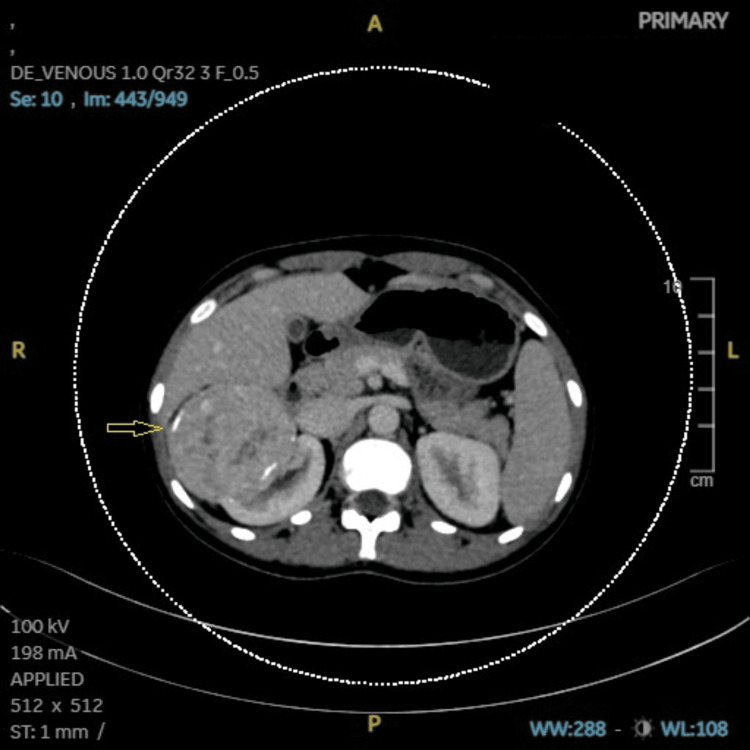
Case 4: CECT (abdomen and pelvis) showing large (9.5 × 7 × 6 cm) right renal mass with peripheral curvilinear calcification (arrow). CECT: contrast-enhanced computed tomography, A: anterior, R: right, L: left, P: posterior, ST: soft tissue, WW: window width, WL: window level.
